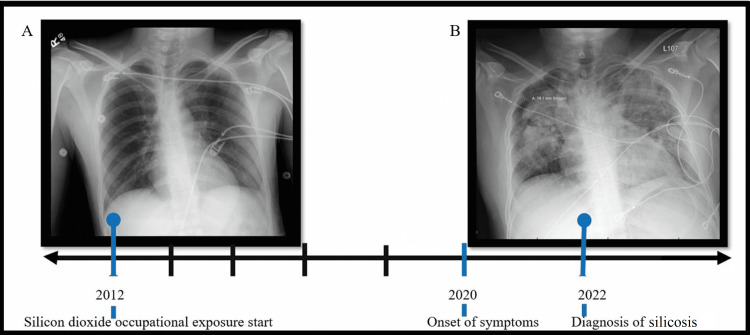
Chest x-ray transition regarding accelerated silicosis. The image shows (A) the chest x-ray 10 years prior and (B) the chest x-ray at the time of diagnosis.
